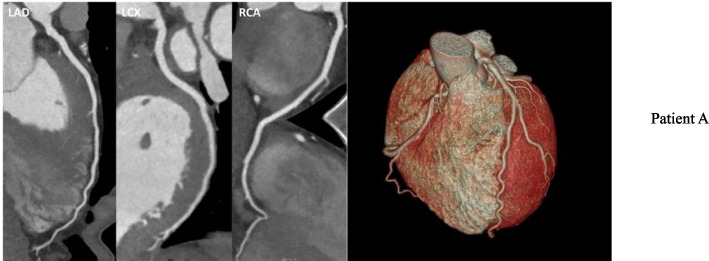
Coronary CT angiography demonstrating normal coronary arteries. This figure presents a representative coronary CT angiography (CCTA) image from a patient experiencing 5-FU-related chest pain (Patient A). Due to the presence of regional hypokinesis on echocardiography, CCTA was utilized to rule out significant coronary stenosis or plaque rupture. Results showed patent epicardial coronary arteries, confirming that anginal symptoms were caused by functional vasospasm rather than structural obstruction. This exclusion is critical for safely initiating antianginal treatment before reintroducing chemotherapy*. Abbreviations: CCTA: coronary CT angiography; 5-FU: 5-fluorouracil*
